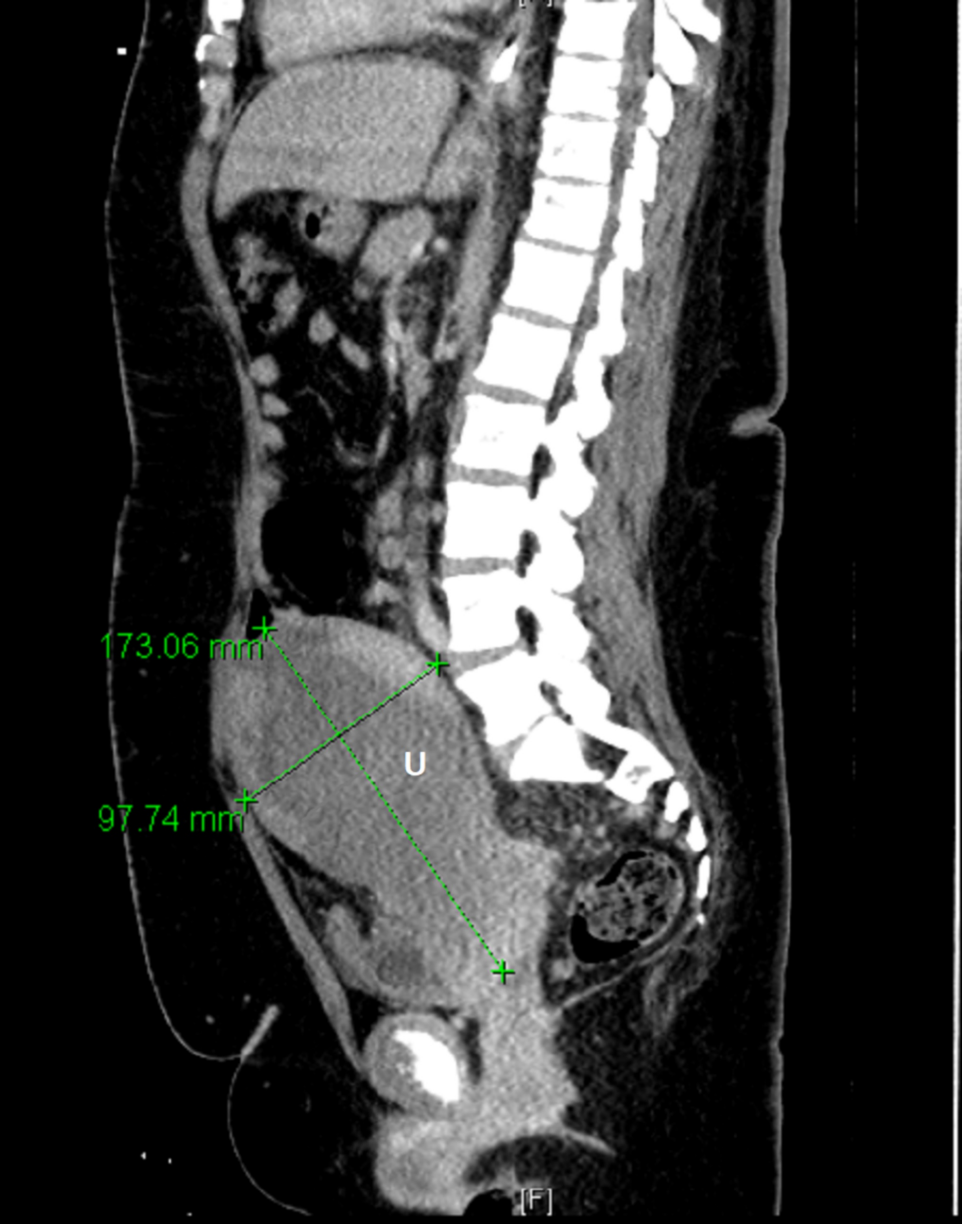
Sagittal view of CT abdomen and pelvis with intravenous contrast, showing large heterogeneous uterine mass (U) CT: computed tomography
